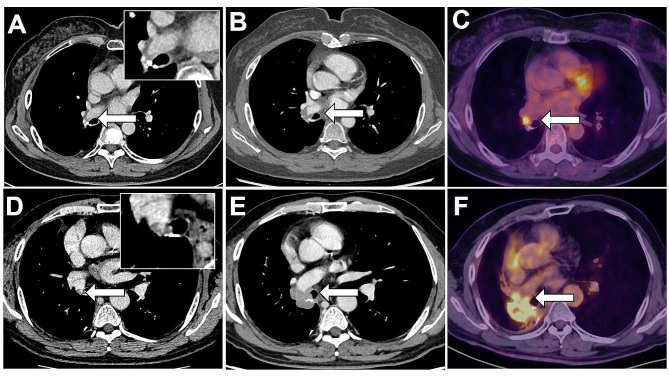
The malignant progression of concave-shaped pulmonary arterial stump filling defects. (A) A 64-year-old female lung cancer patient exhibiting a concave-shaped filling defect in the pulmonary arterial stump on CT 134 days post-lobectomy. (B) Progressive increase in the filling defect observed 65 days post-onset. (C) Corresponding PET-CT image of highlighting fluorodeoxyglucose concentration in the filling defect. (D) A 63-year-old male lung cancer patient displaying a oncave-shaped filling defect in the pulmonary arterial stump on CT 222 days post-lobectomy. (E) Following anticoagulant therapy with Rivaroxaban, the follow-up CT scan revealed an increased filling defect and the recurrence of the vascular stump 180 days post-onset. (F) Corresponding PET-CT image of showing fluorodeoxyglucose concentration in both the recurrent neoplasm and the filling defect
